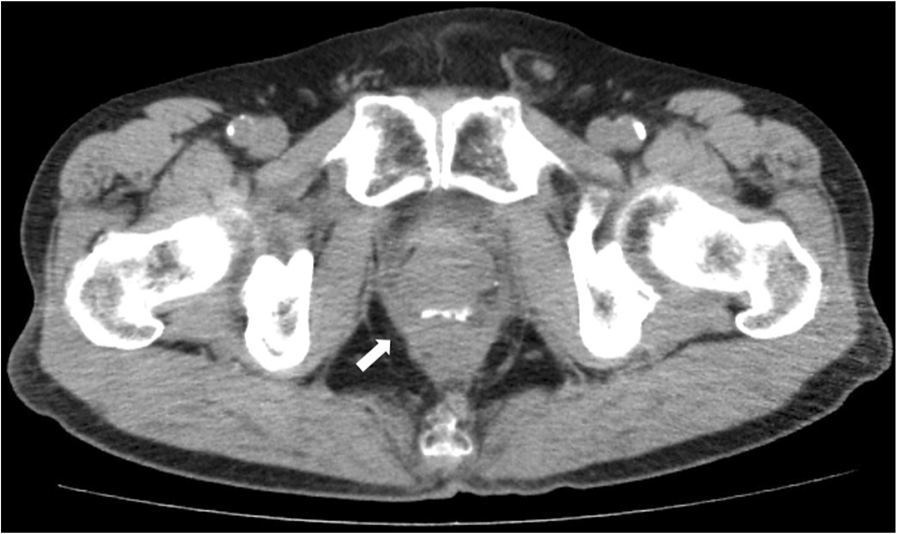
Computed tomography scan 3 months before the patient presented with symptoms of a leak shows a circumferential staple line at the anastomotic site
